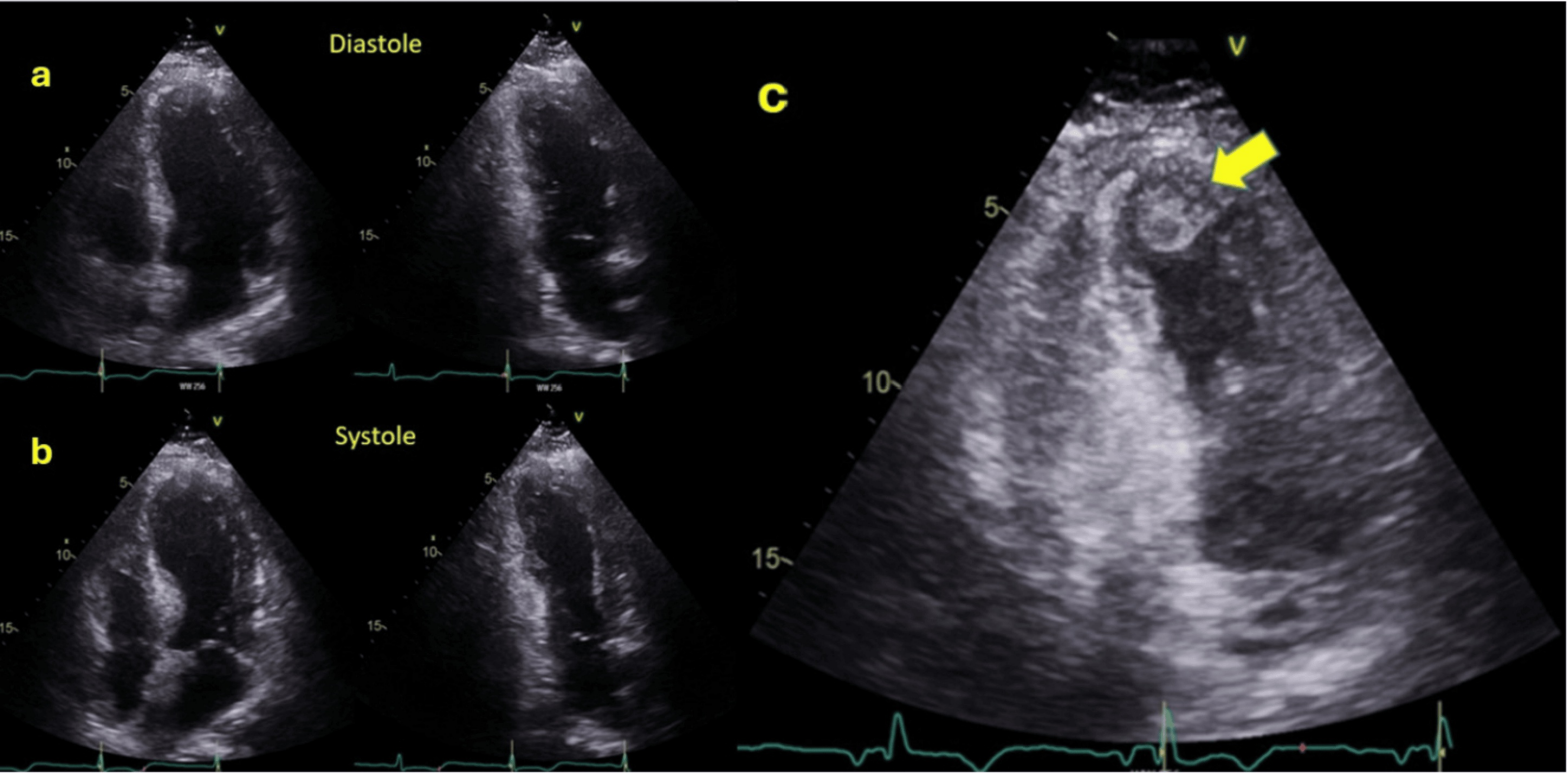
Echocardiogram performed 24 hours after PCI showing moderate left ventricular systolic dysfunction with akinesia of the mid-apical segments and LV apical trombus (yellow arrow) a: LV diastole; b: LV systole, revealing akinesia of the mid-apical segments; c: LV apical thrombus (yellow arrow) PCI: Percutaneous coronary intervention, LV: Left ventricle
